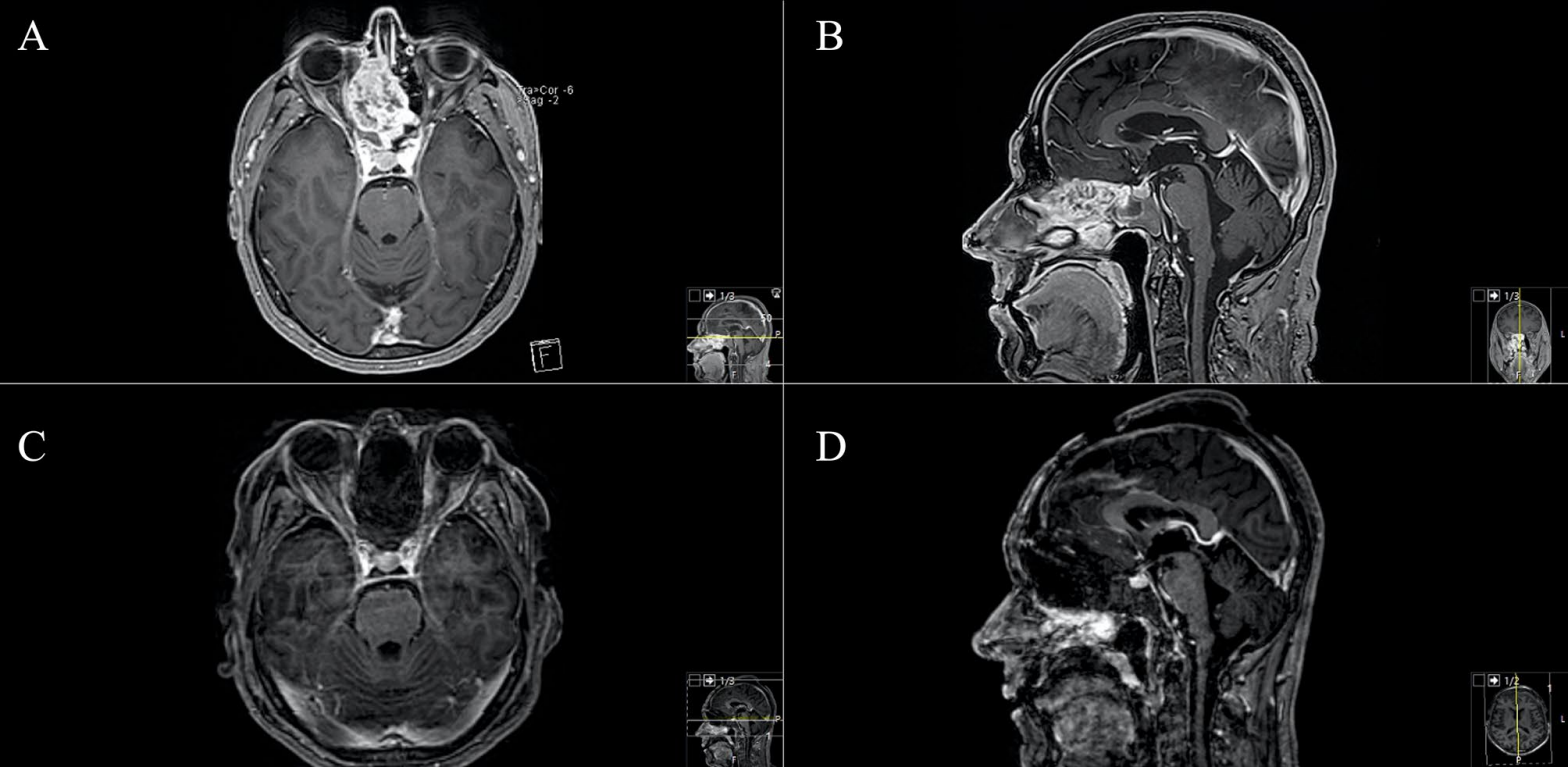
Exemplary case 5: a 64-year-old male patient with anosmia, rhinorrhoea, epiphora and headache. Transnasal biopsy revealed sinusinal intestinal adenocarcinoma. (A,B) Preoperative T1-weighted gadolinium-enhanced MR imaging endonasal tumor with transbasal infiltration into sphenoid sinus, cribriform plate and dislocation of right periorbit. (C,D) Postoperative MR control showing subtotal resection with questionable tumor remnant at inferior medial orbital wall. Tumor-infiltrated medial orbital walls and orbit roofs were resected. Reconstruction was performed with split-bone graft and galea-periosteum flap. Titan mesh was used as well. A second-step endoscopic procedure was performed for oncological gross total resection.
